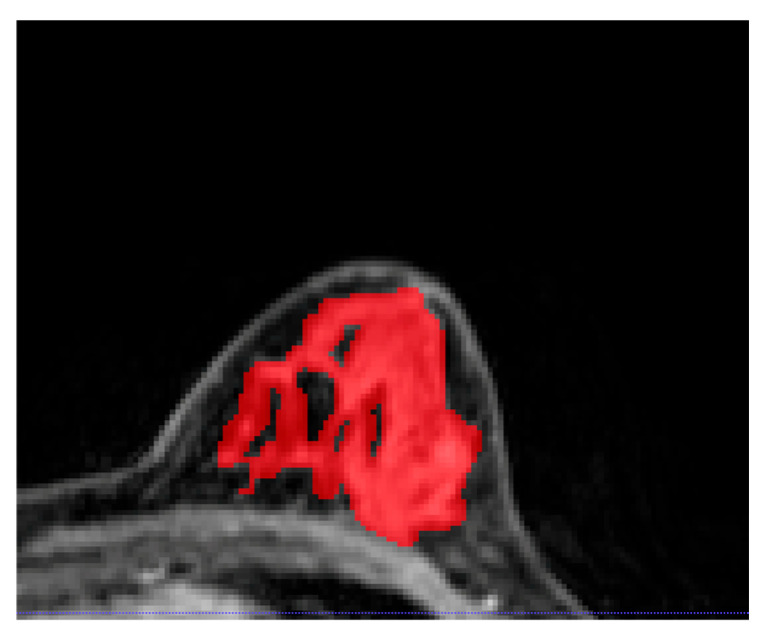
An example of fibroglandular tissue segmentation from the contralateral healthy breast on post-contrast T1-weighted imaging. Interspersed as well as surrounding subcutaneous adipose tissue were excluded from the segmentation.
